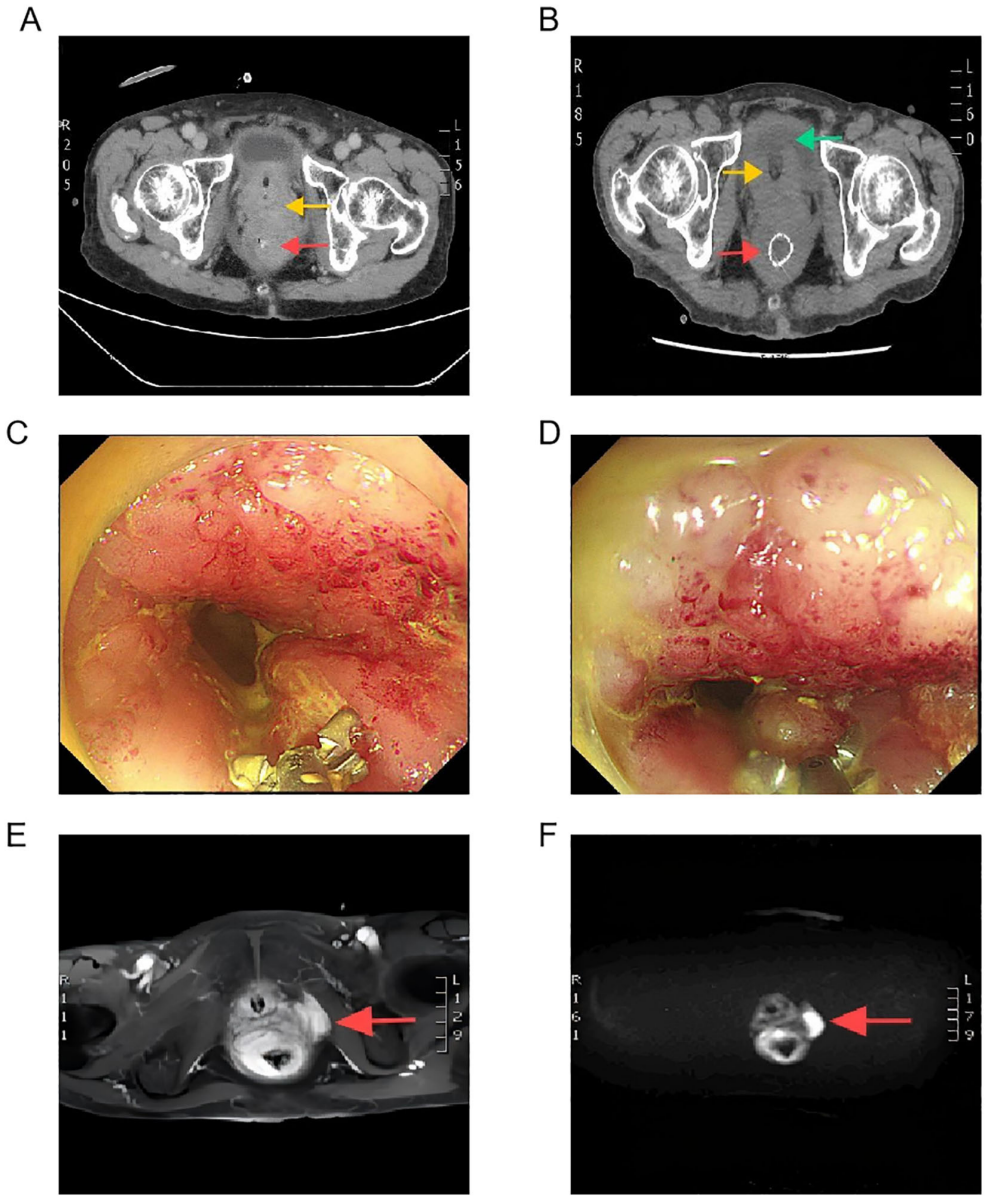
Results of imaging studies and endoscopic examination. (A) Contrast-enhanced CT of the lower abdomen.The red arrow indicates: thickened rectal wall; the yellow arrow indicates: prostate-rectal junction. (B) Non-contrast 64-slice CT of the lower abdomen. The red arrow indicates a thickened rectal wall; the yellow arrow indicates the prostate; the green arrow indicates the bladder. (C, D) Electron colonoscopy revealed rectal edema and luminal obstruction. (E) T1-weighted imaging (T1WI) sequence with contrast enhancement. The encircled region represents the lesion at its maximum dimension. (F) Diffusion-weighted imaging (DWI) sequence. The encircled region represents the lesion at its maximum dimension.
